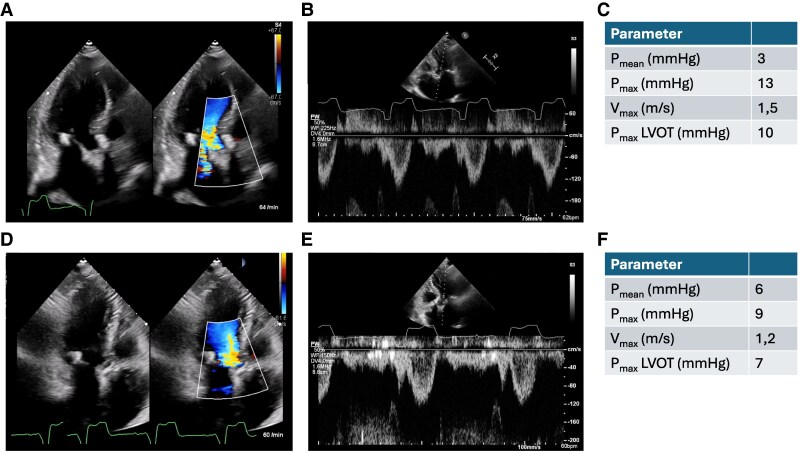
Apical three-chamber view, pulse wave Doppler in the left ventricular outflow tract and Doppler over the aortic valve 10 days after transcatheter aortic valve implantation (*A–C*) and 9 weeks after transcatheter aortic valve implantation (*D–F*).
